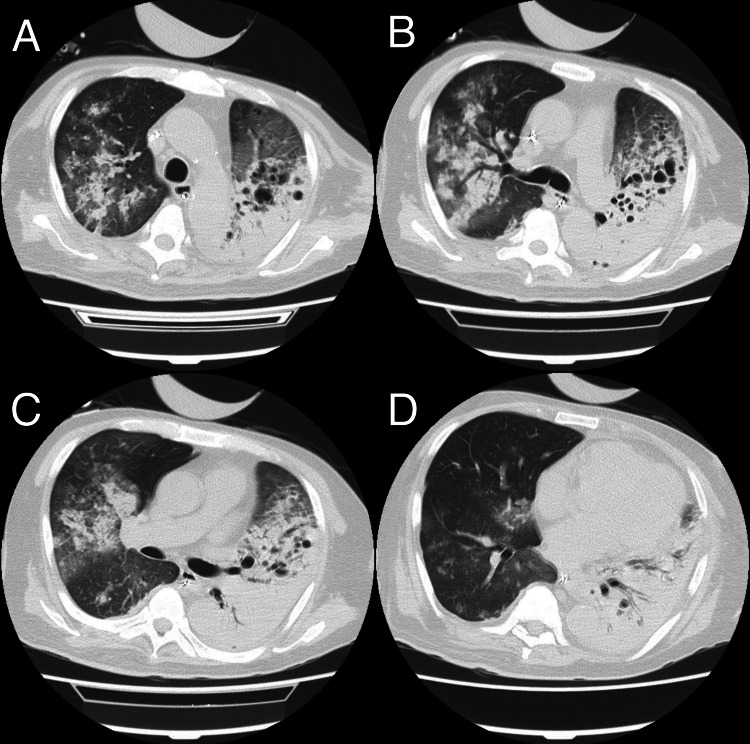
Chest CT on day 13 showing resolution of pulmonary infiltrates in ARDS in TB Chest CT images on day 13 demonstrate partial resolution of consolidations, though bilateral consolidations and multiple cavitary lesions in the left lung are still present (A, B, C, and D). CT: computed tomography, ARDS: acute respiratory distress syndrome, TB: tuberculosis
